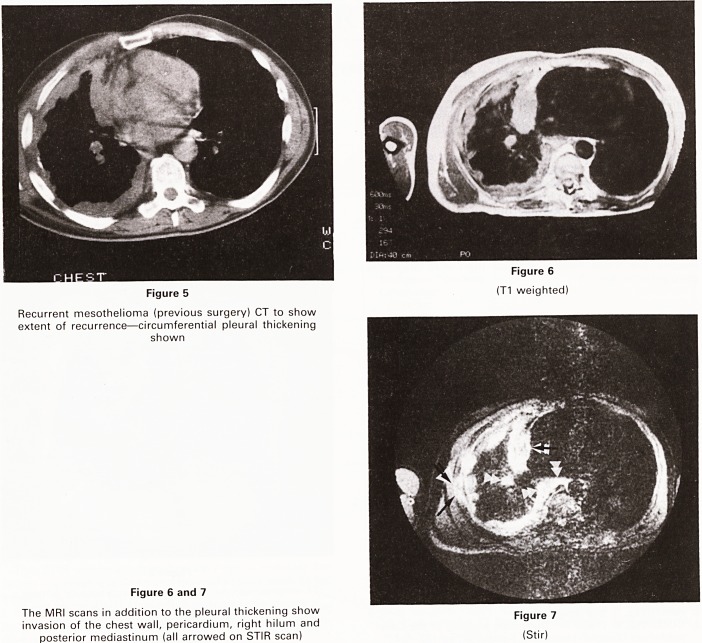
# MRI in Thoracic Malignancy
